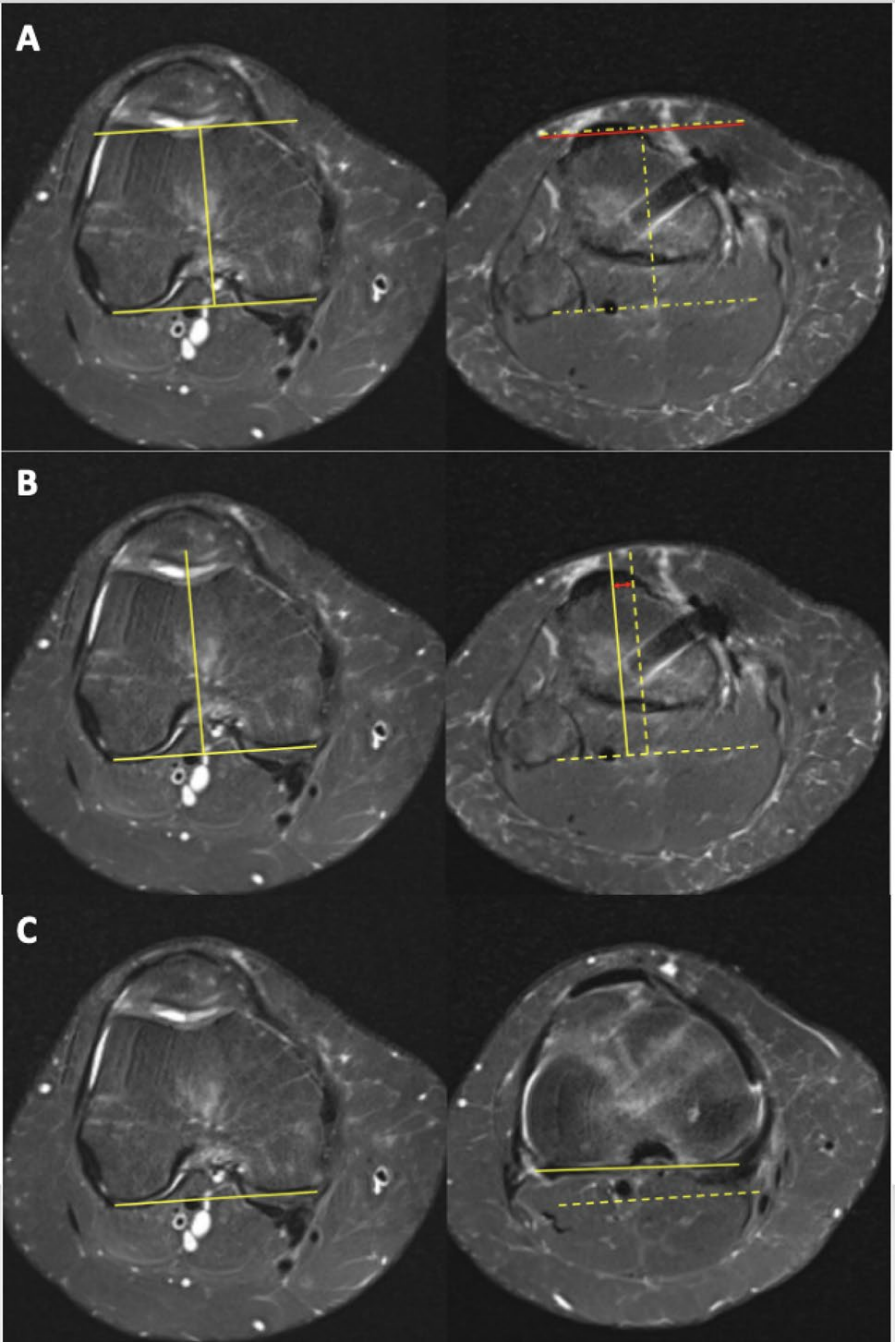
MRI-based measurement techniques. A Sagittal tibial tubercle–trochlear groove (sTT–TG) distance: The deepest point of the trochlear groove was identified on the axial slice showing the Roman arch, and a line perpendicular to the posterior femoral condylar axis was drawn (left panel, yellow lines). The most prominent point of the tibial tubercle was located, and its anteroposterior distance relative to the trochlear groove was measured (right panel, vertical red line to the yellow line). A positive value indicated a posteriorly positioned tibial tubercle. B Tibial tubercle–trochlear groove (TT–TG) distance: The mediolateral distance (red double arrow) between the trochlear groove and the tibial tubercle was measured on axial images, using perpendicular lines referenced to the posterior condylar axis (yellow lines). C Tibiofemoral rotation angle (TFRA): Defined as the angle between the posterior femoral condylar axis (yellow line, left) and the posterior tibial axis just distal to the tibial plateau (yellow line, right). A positive angle indicated internal tibial rotation relative to the femur
